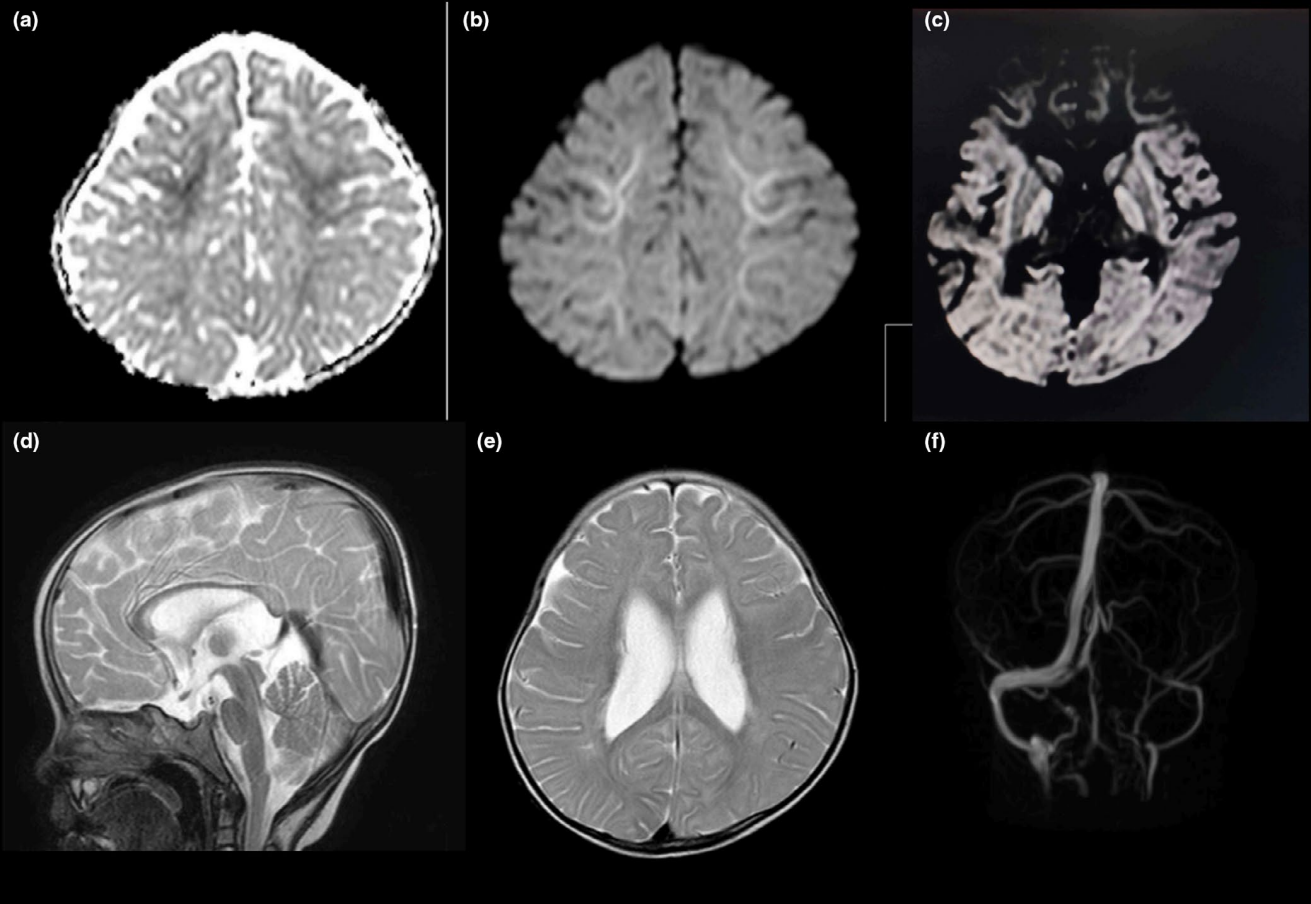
Magnetic resonance imaging (MRI) of the brain. (a) Apparent diffusion coefficient (ADC) showing mild restricted diffusion in subcortical white matter of Frontal Lobe (ADC is a measure of the magnitude of diffusion of water molecules within tissue, and is calculated using MRI with diffusion‐weighted imaging (DWI). (b) Diffusion‐weighted imaging showing mild restricted diffusion in subcortical white matter of Frontal Lobe. (c) Diffusion weighted imaging showing mild restricted diffusion in subcortical white matter of bilateral parietal lobe and globus pallidus. (d) T2 weighted MRI showing aplastic frontal (arrows) and sphenoid paranasal sinuses. (e) T2 weighted MRI showing mild white matter volume loss on the periventricular area of the lateral ventricle. T1 and T2 are basic pulse sequences on a MRI. A T1 MRI image supplies information about current disease activity by highlighting areas of active inflammation. A T2 MRI image provides information about disease burden or lesion load (the total amount of lesion area, both old and new). (f) Magnetic resonance (MR) angiogram showing aplasia of left transverse and sigmoid dural venous sinus.
